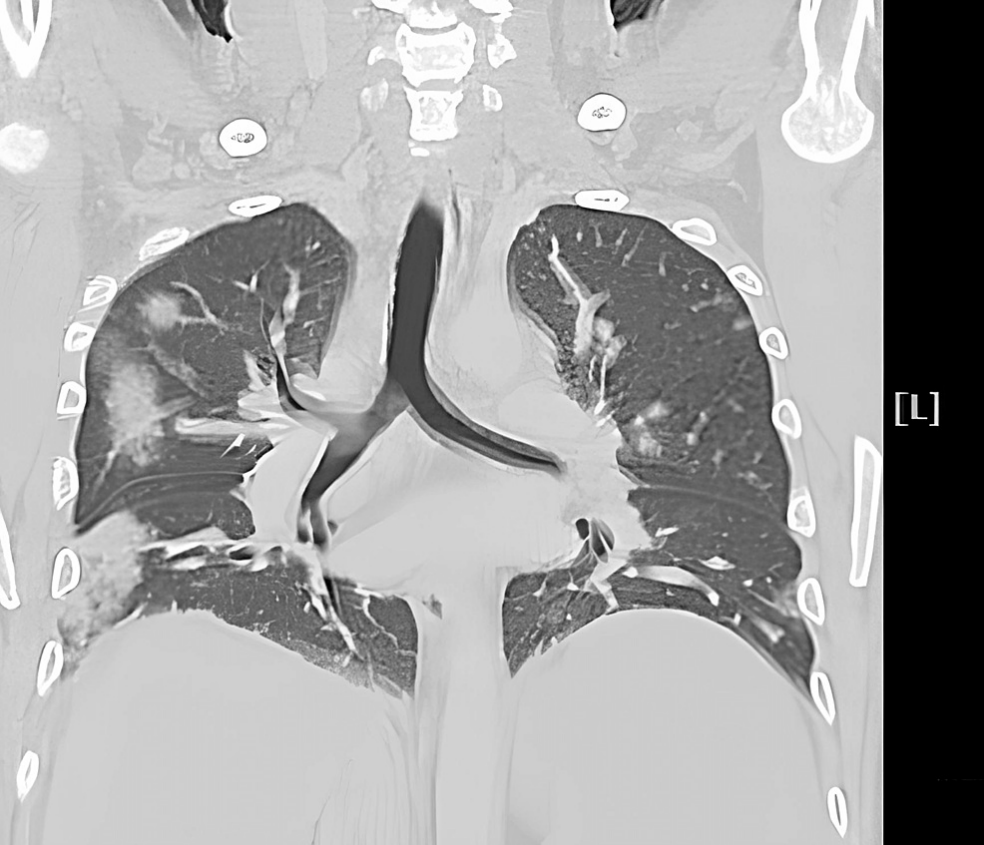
The computed tomography of the thorax showed multiple nodular and patchy infiltrates at right lung, and septic pulmonary embolism could be identified.
